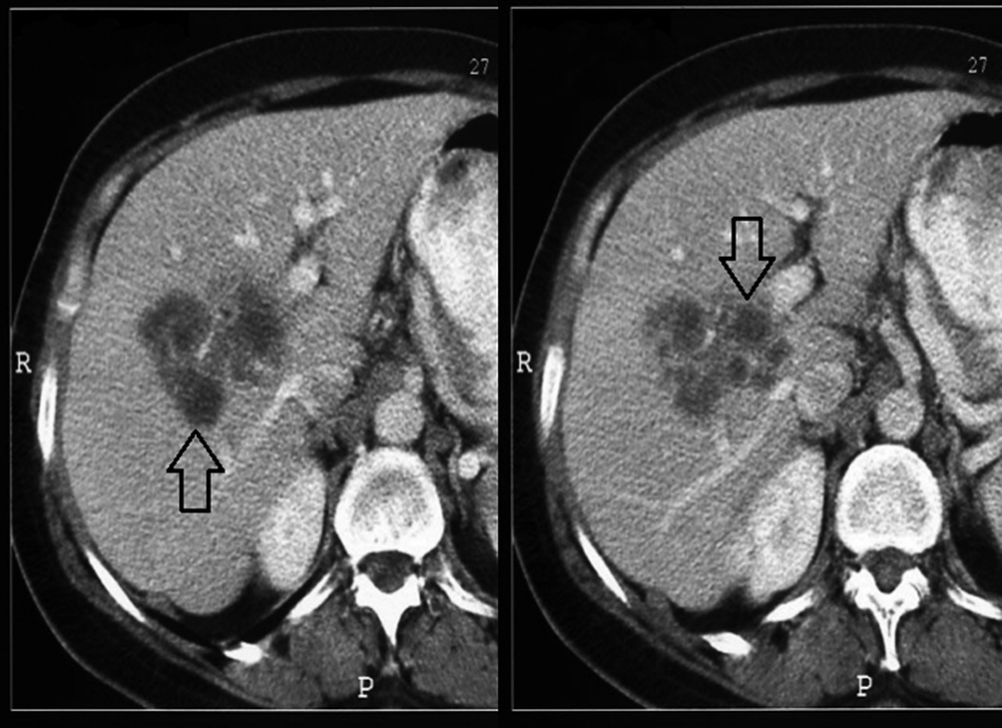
Contrast-enhanced CT images in the portal phase show multiple small, clustered hypodense nodular masses with hazy irregular margins in the right lobe of the liver parenchyma (arrows).
